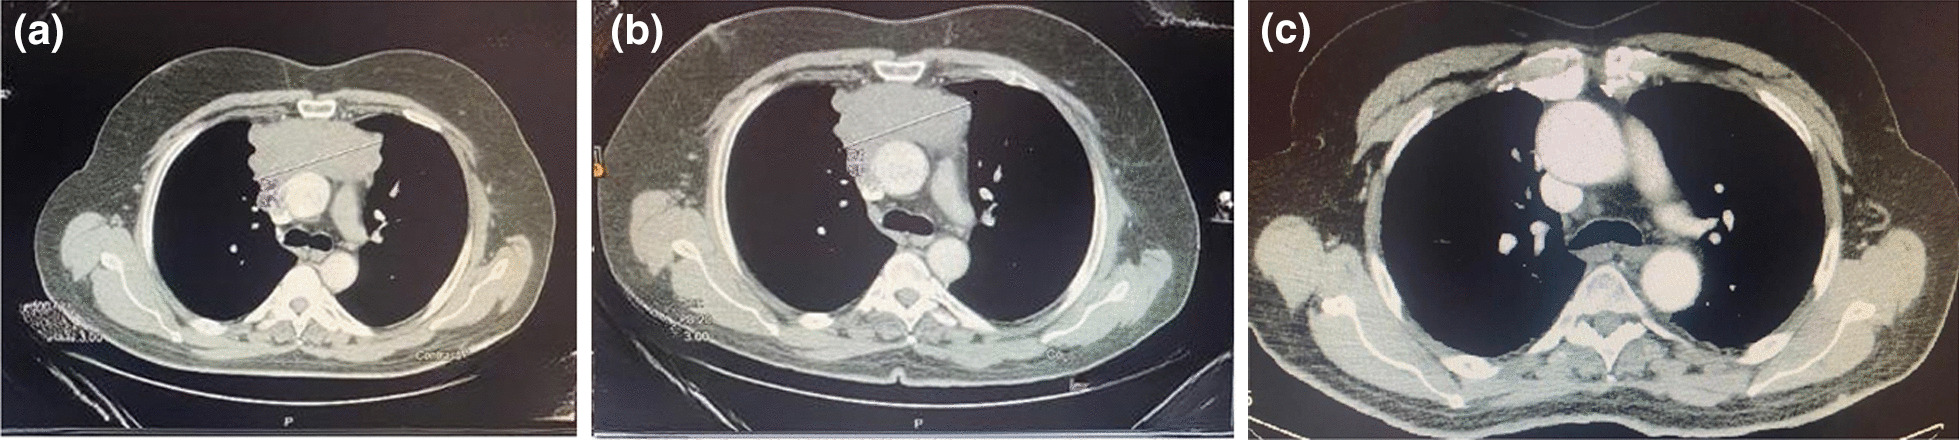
a Computed tomography (CT) of thymoma tumor pre-neoadjuvant chemotherapy. b Computed tomography (CT) of thymoma tumor post-neoadjuvant chemotherapy.  c Chest computed tomography (CT) after surgery
